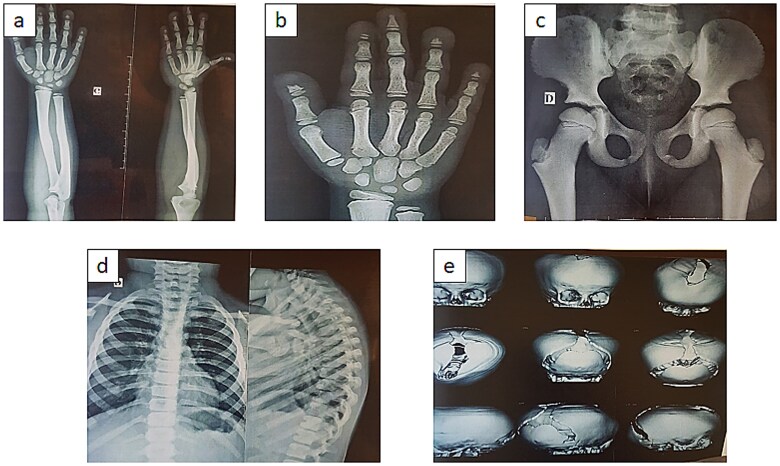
Skeletal X-rays. a. X-ray of the forearm, front and side view, showing bone hyperdensity of the radius, ulna, and metacarpals; b. X-ray of the hand showing acro-osteolysis of the distal phalanges of the 1st, 2nd, 4th, and 5th fingers with short, stocky metacarpals; c. frontal pelvic X-ray showing diffuse osteosclerosis and marked thickening of the pelvic and femoral cortex with a bilateral jagged appearance of the iliac wings; d. front and side X-ray of the spine showing scoliosis and kyphosis of the thoracic spine with vertebral bone hyperdensity; e. skull X-ray showing diffuse osteosclerosis with bone hyperdensity, widening of the sutures, delayed closure of the fontanelles, and wormian bones.
